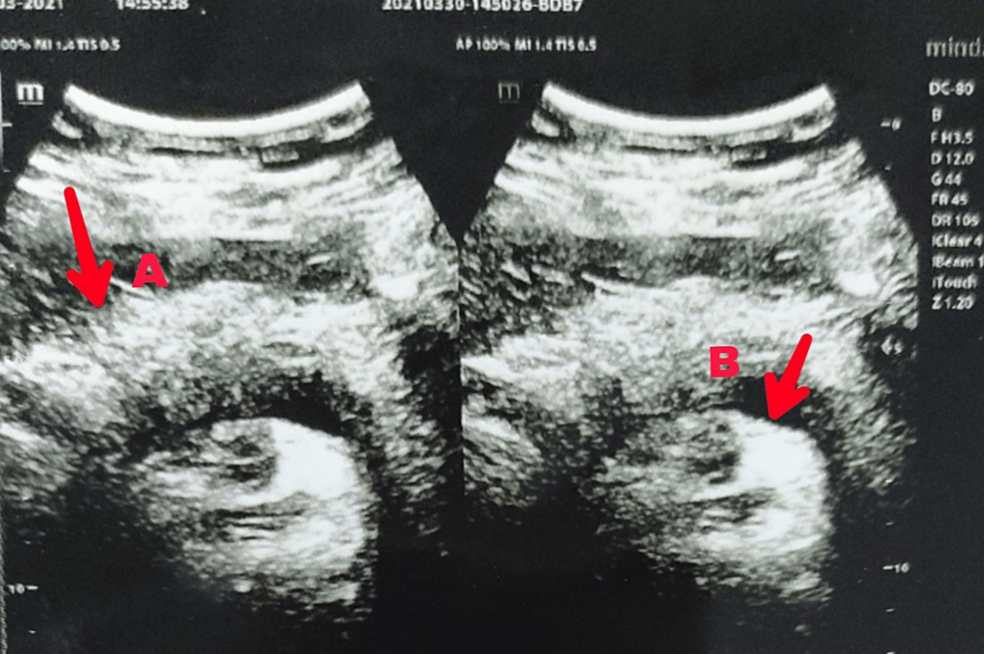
Abdominal ultrasound scan shows a thickened wall of gall bladder (A) and hyperechoic cystic cavity (B) filled with sludge-like fluid. Liver appears to be pale.
